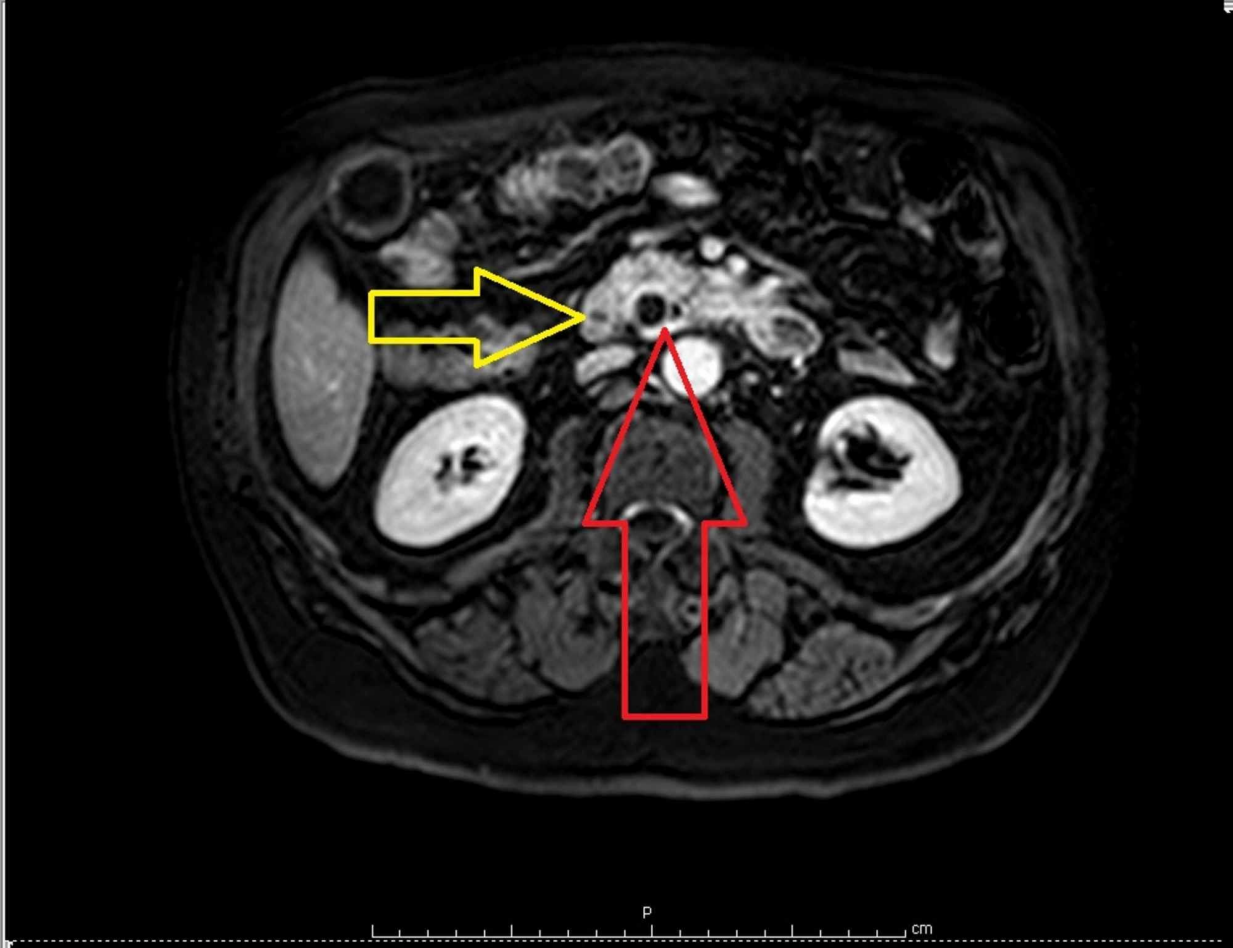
Abdominal magnetic resonance imaging (MRI). The red arrow indicates the dilatation of the common bile duct (CBD) and major pancreatic duct (MPD) caused by the endoluminal duodenal mass (yellow arrow).
